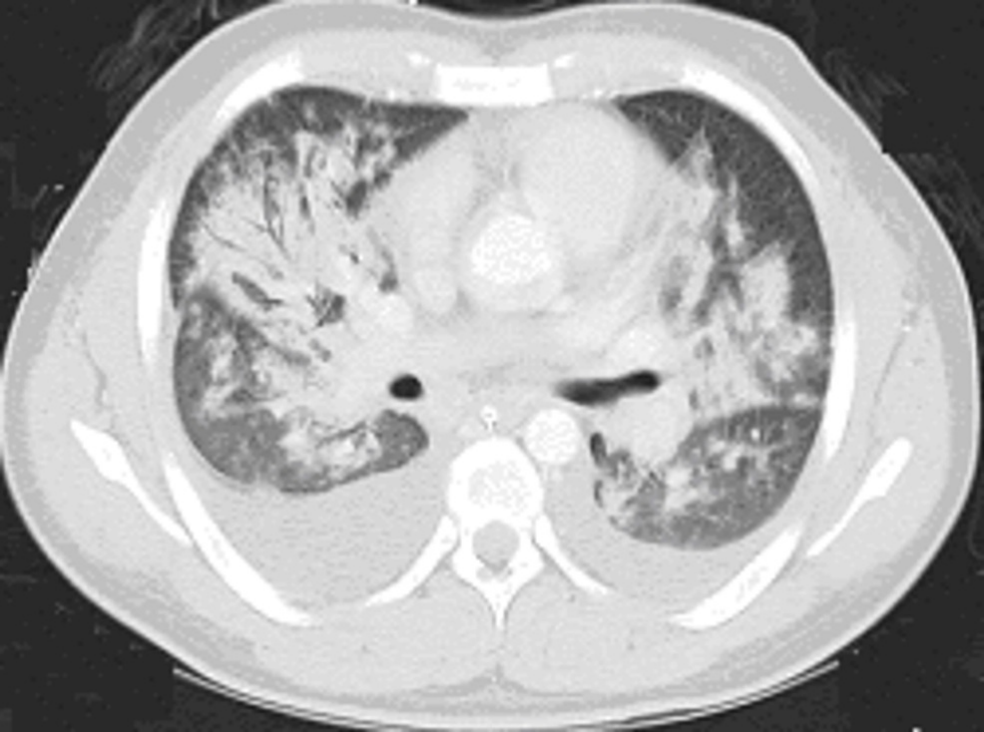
CT chest with IV contrast Axial chest CT demonstrates multifocal pneumonia throughout both lungs and bilateral pleural effusions CT: computed tomography; IV: intravenous
